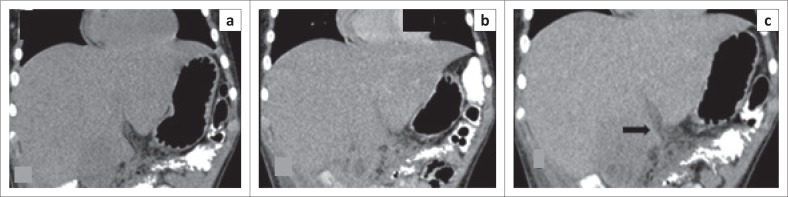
A 36-year-old female who presented with a long history of intermittent fever. Coronal computed tomography scan images of an abscess in segment V of the liver (a) precontrast, (b) arterial phase and (c) portovenous phase showing incomplete ring enhancement (arrow).
